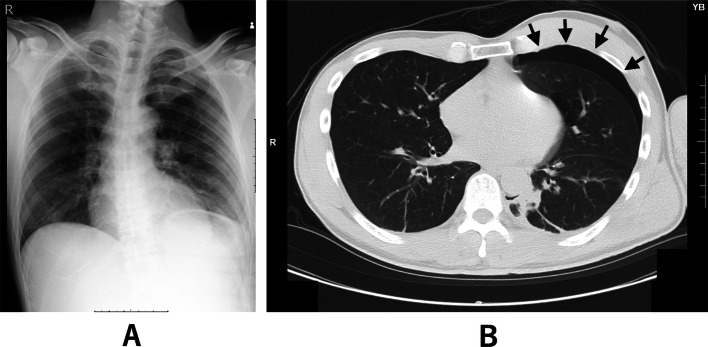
Chest radiography (A) showed no obvious pneumothorax, while computed tomography (B) revealed a mild left-sided pneumothorax
